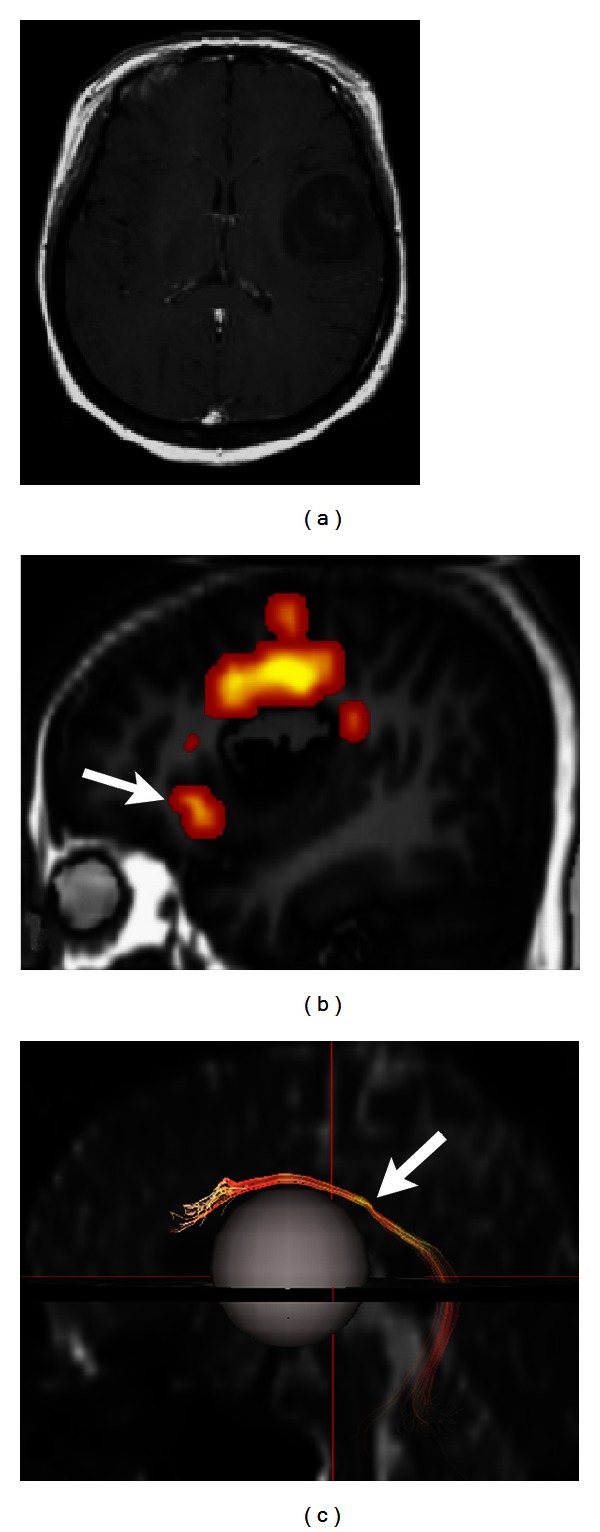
(a) Contrast-enhanced T1-weighted image shows an oligodendroglioma, WHO grade II, in the left frontal lobe. (b) Functional MR imaging and DTI tractography (c) demonstrate the activation of Broca's area (arrow in (b)) anterior to the tumor and the elevated arcuate fasciculus (arrow in (c)), respectively. The grey sphere in Figure 5(c) indicates the location of tumor.
